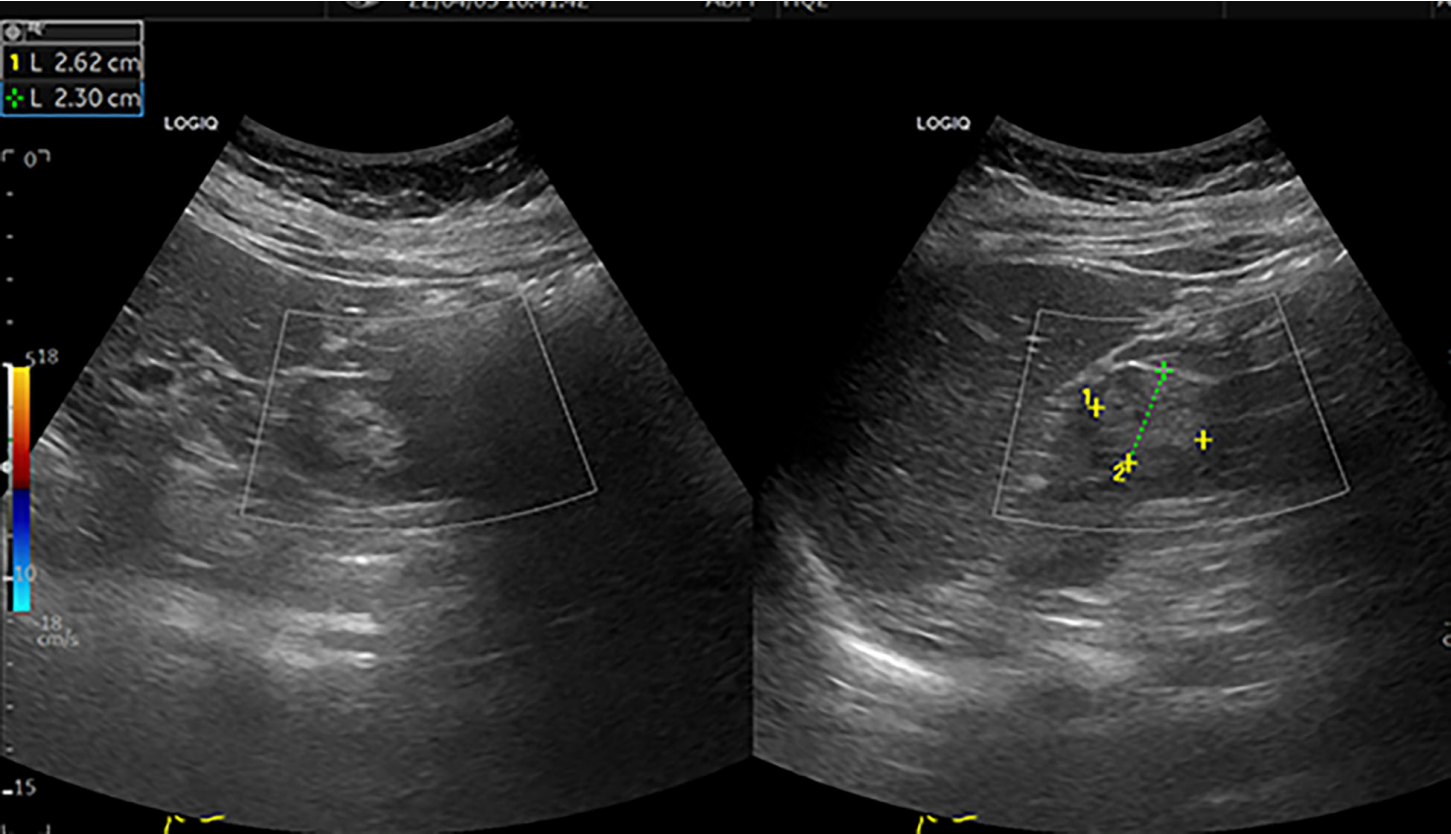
Colour ultrasound. This image shows a moderate echogenic mass of approximately 2.6 cm×2.3 cm in the middle and upper parts of the left kidney, with a clear boundary and regular shape [yellow^+^ and green^+^]. CDFI: no blood flow signal in the mass.
